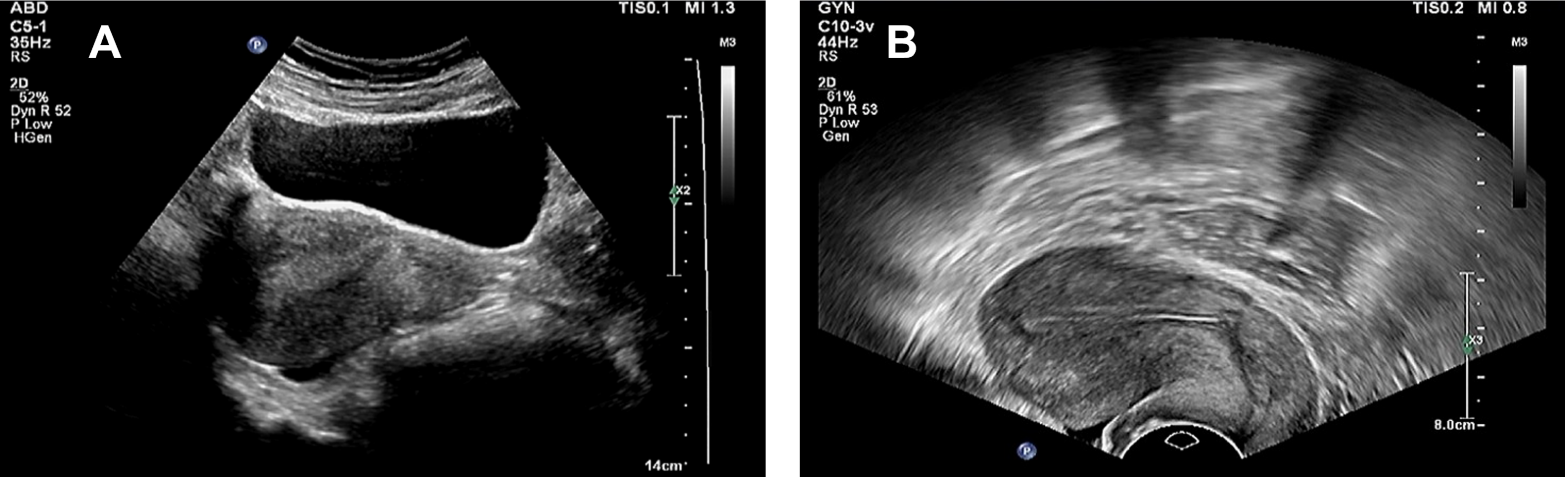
Endometrial ultrasound images of typical cases. (A) Heterogeneous echo (EC patient. (B) Homogeneous echo (patients with benign lesions).
